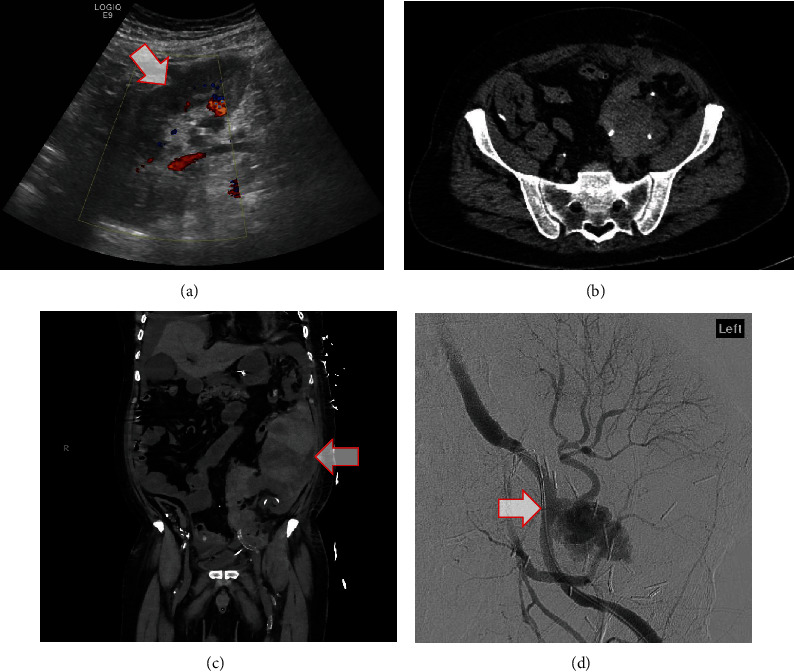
Case 1 imaging, whose blood and urine cultures on admission grew *Pseudomonas aeruginosa*. (a) Ultrasound on admission demonstrating normal LLQ transplant kidney with patent renal artery and vein without pseudoaneurysm. (b) Noncontrast CT A/P demonstrating slight interval increase in size of hematoma compared to prior imaging. No evidence of active bleeding. (c) CT angiogram demonstrating irregular, actively bleeding pseudoaneurysm arising from the transplant renal artery ~1.2 cm from the anastomosis. (d) Arteriogram demonstrating pseudoaneurysm with active contrast extravasation. A 6 mm × 22 mm covered stent was placed successfully within the proximal transplant renal artery; however, persistent hemorrhage warranted emergent surgical management.
